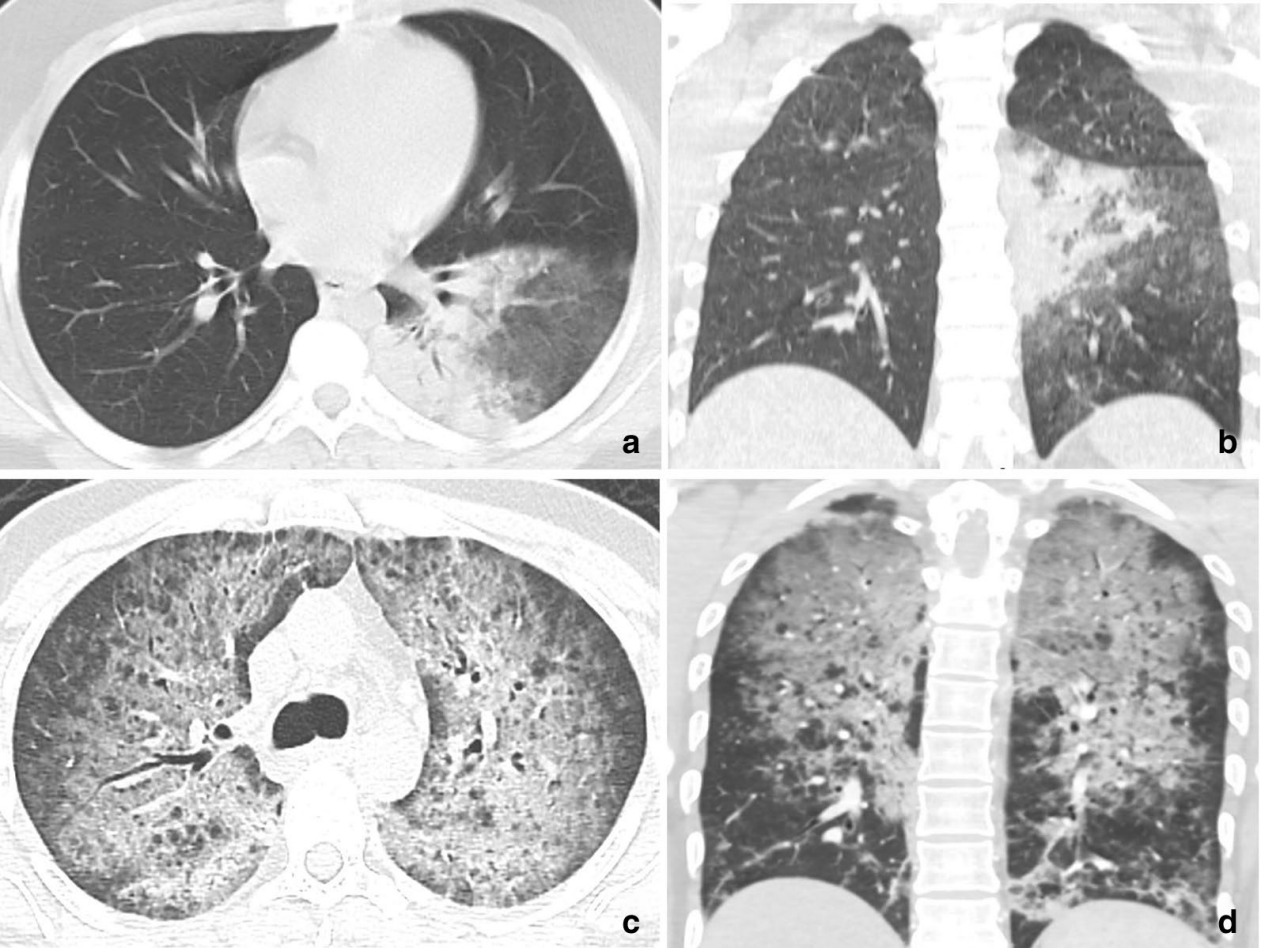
Atypical infection mimicking indeterminate COVID-19 and viral pneumonia mimickers. a, b A 39-year-old man with Mycoplasma pneumonia, axial and coronal reformatted CT images shows unilateral lobar consolidation with nearby GGO and centrilobular nodules. c, d Pneumocystis jirovecii pneumonia in a 36-year-old man with positive HIV test, axial and coronal reformatted CT images show extensive bilateral ground-glass opacities with relative subpleural sparing
